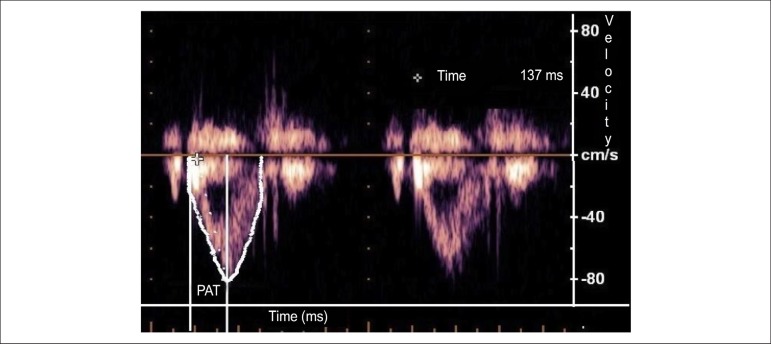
Pulsed-wave Doppler of pulmonary artery (PAT: pulmonary acceleration
